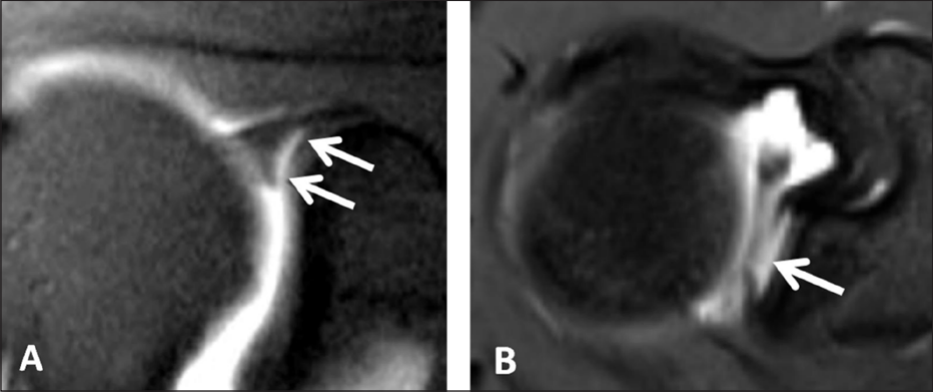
Sublabral recess (sublabral sulcus). (A) Coronal oblique fat-suppressed T1-weighted MR arthrographic image shows a sublabral recess as an increased linear signal undercutting the contour of the superior glenoid labrum (arrows, A) following the contour of the glenoid cartilage without extension posterior to the biceps anchor. This sulcus is visualized on (B) Axial T1-weighted MR arthrographic image (arrow, B) on an upper section.
